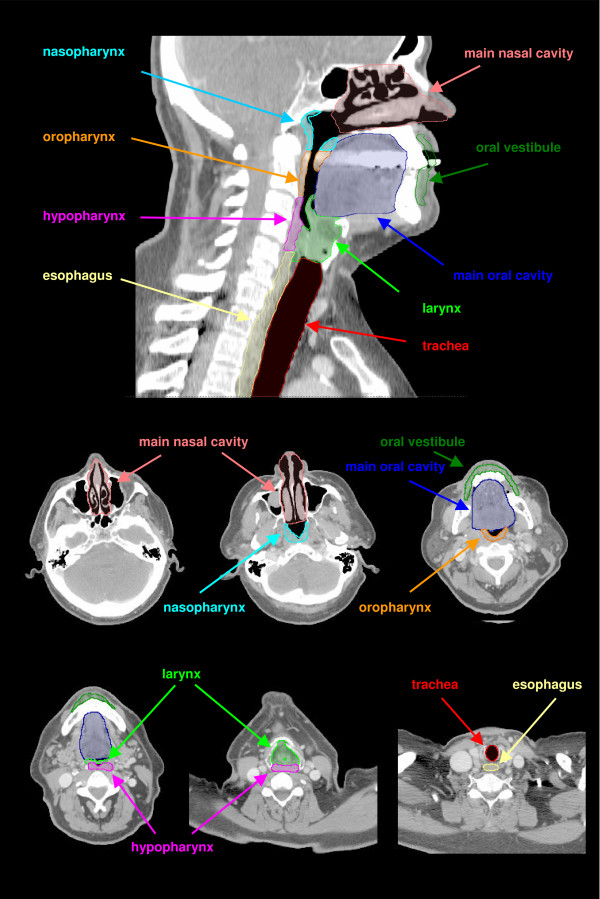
Anatomic head and neck regions contoured on a sagittal DRR and transversal CT slices.
